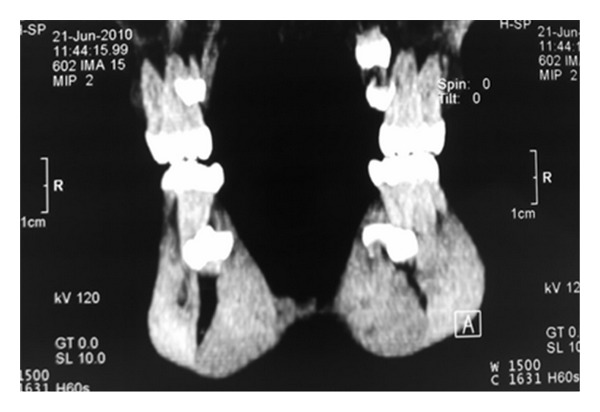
CT scan to evaluate the position of supernumeraries.
